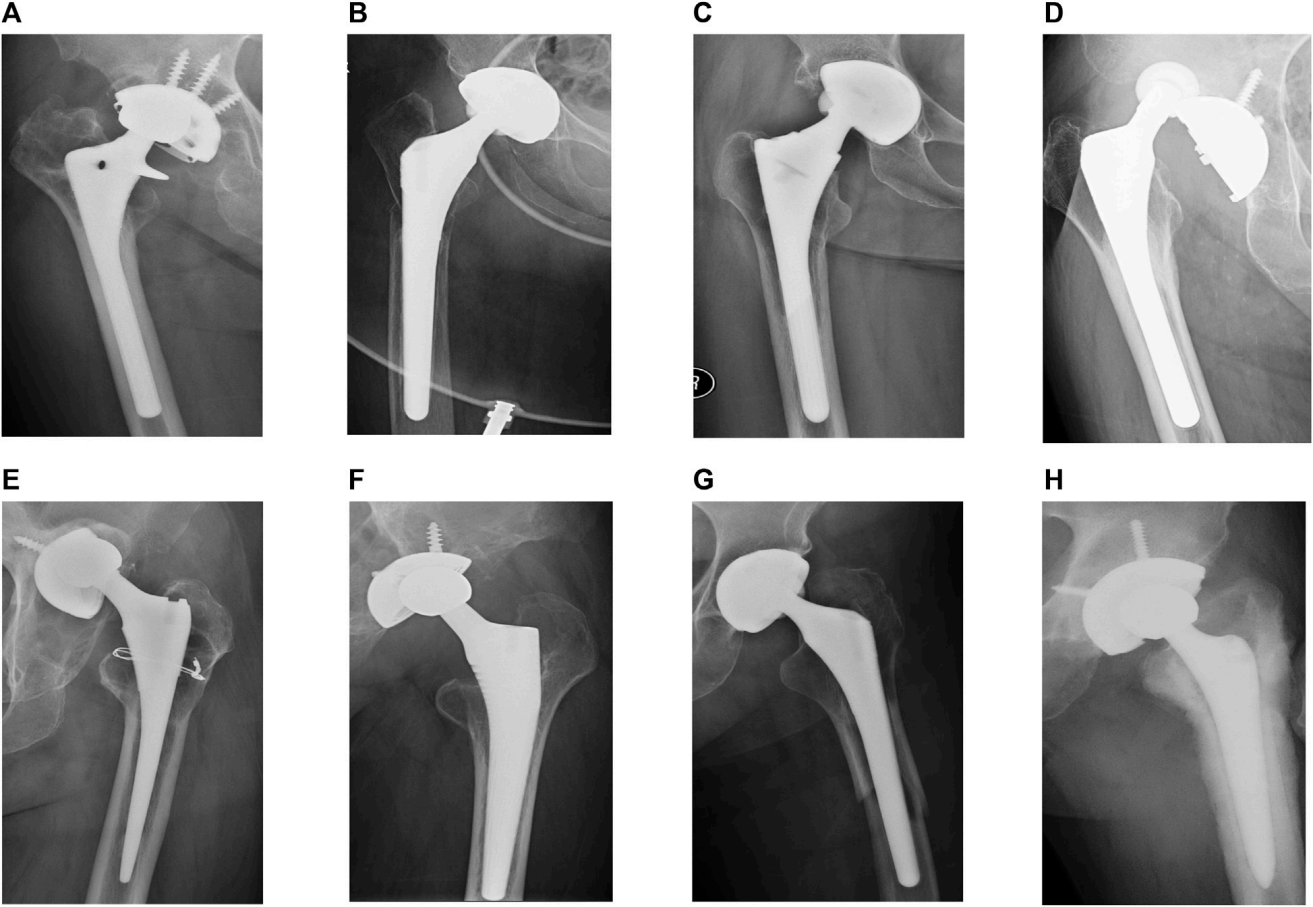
Examples of X-ray images of complications after total hip arthroplasty (THA) in our dataset cropped by our own trained YOLO-v5 model to preserve the periprosthetic images. Corresponding labels are labeled by an experienced orthopaedic surgeon as (A) loosening, periprosthetic osteolysis, and prosthetic wear; (B) periprosthetic fracture; (C) loosening; (D) dislocation; (E) loosening, periprosthetic osteolysis, and prosthetic wear; (F) periprosthetic osteolysis, and prosthetic wear; (G) loosening, periprosthetic fracture; and (H) loosening, periprosthetic infection.
